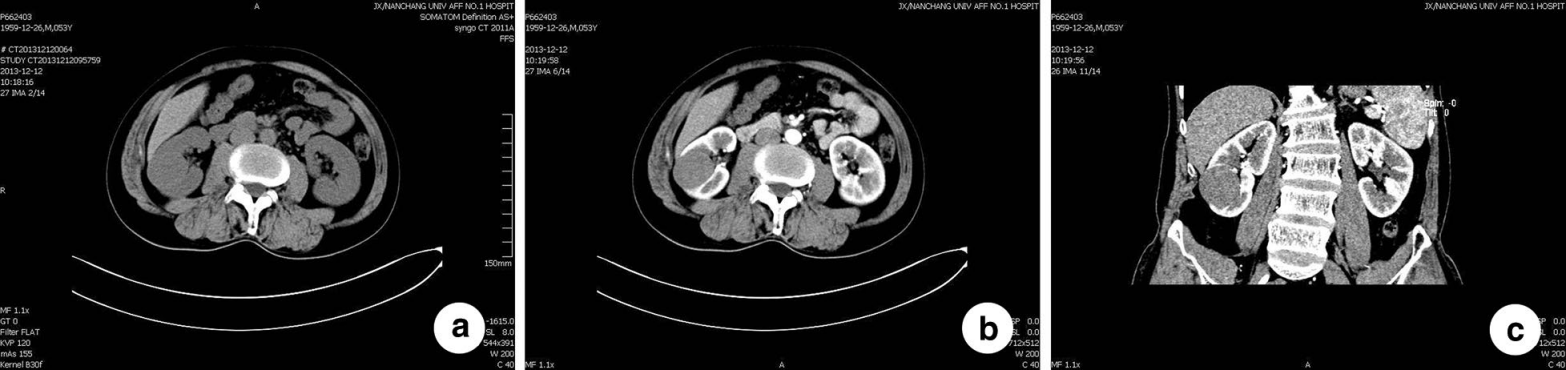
Preoperative CT scan. a Non-contrast CT scan results indicated that there was soft tissue density *shadows* in the lower-middle portion of the *right* kidney, with a clear boundary, a size of 4.2 × 3.8 cm and a CT value of 30–36 HU. b, c The density is uniform, and the enhancement degree of the tumor was significantly lower than that of the surrounding normal renal parenchyma
